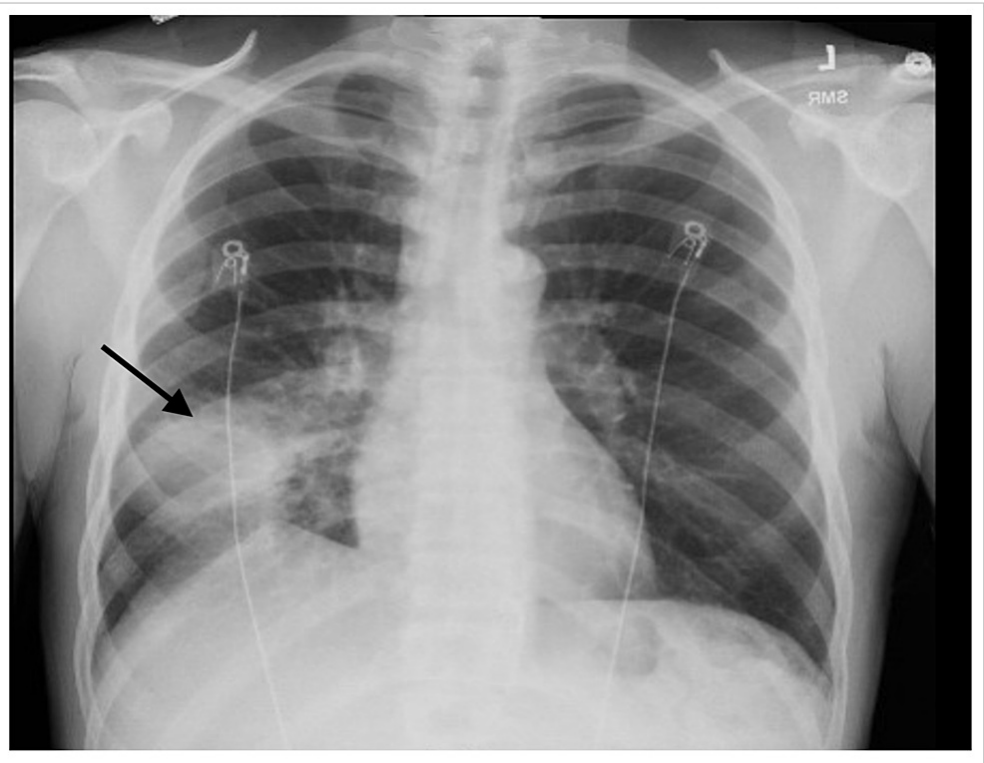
Posteroanterior chest X-ray on admission showing right lower lobe mass (arrow)
